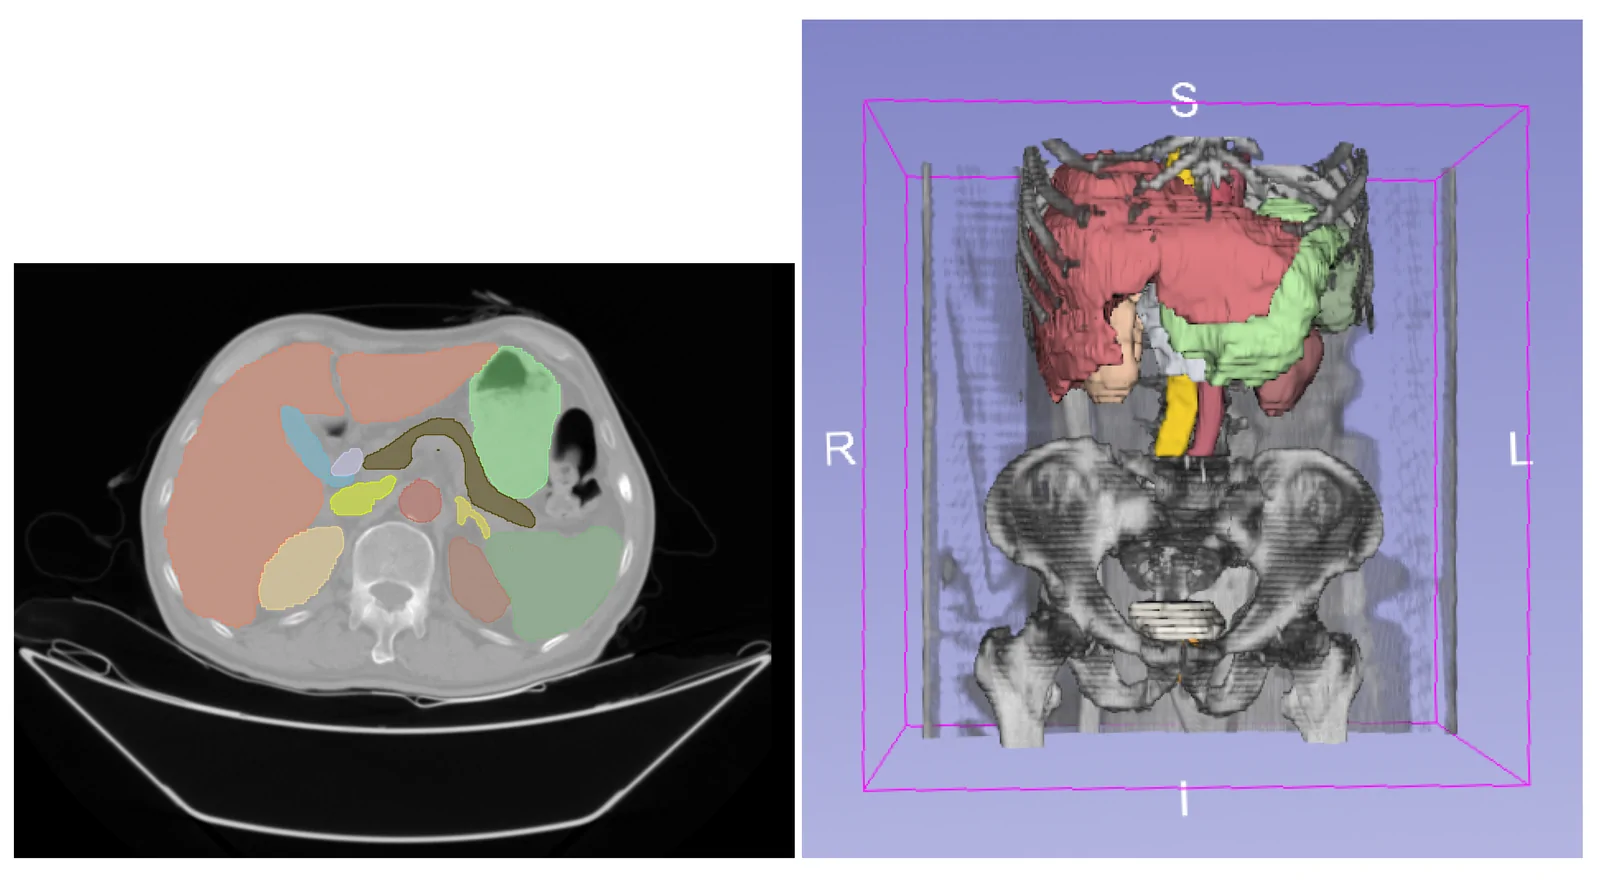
A single sample from the AMOS CT split. (Left): axial slice with per-organ overlay (colour-coded). (Right): 3D volume rendering with the segmentation visible through the body. Both panels were generated in 3D Slicer 5.10.0 [33].
